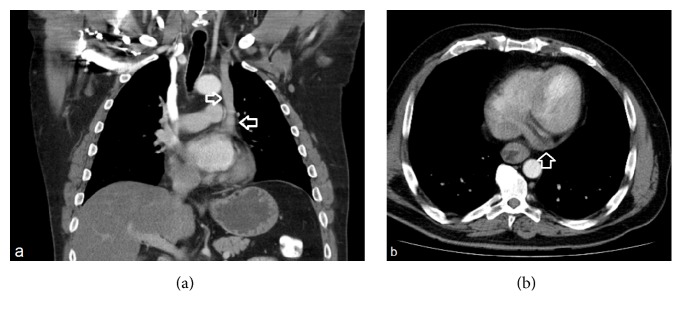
CT of the thorax, including coronal and axial cuts. In picture (a), white arrows show the passage of the PLSSVC while in picture (b) the ending in the coronary sinus is depicted.
